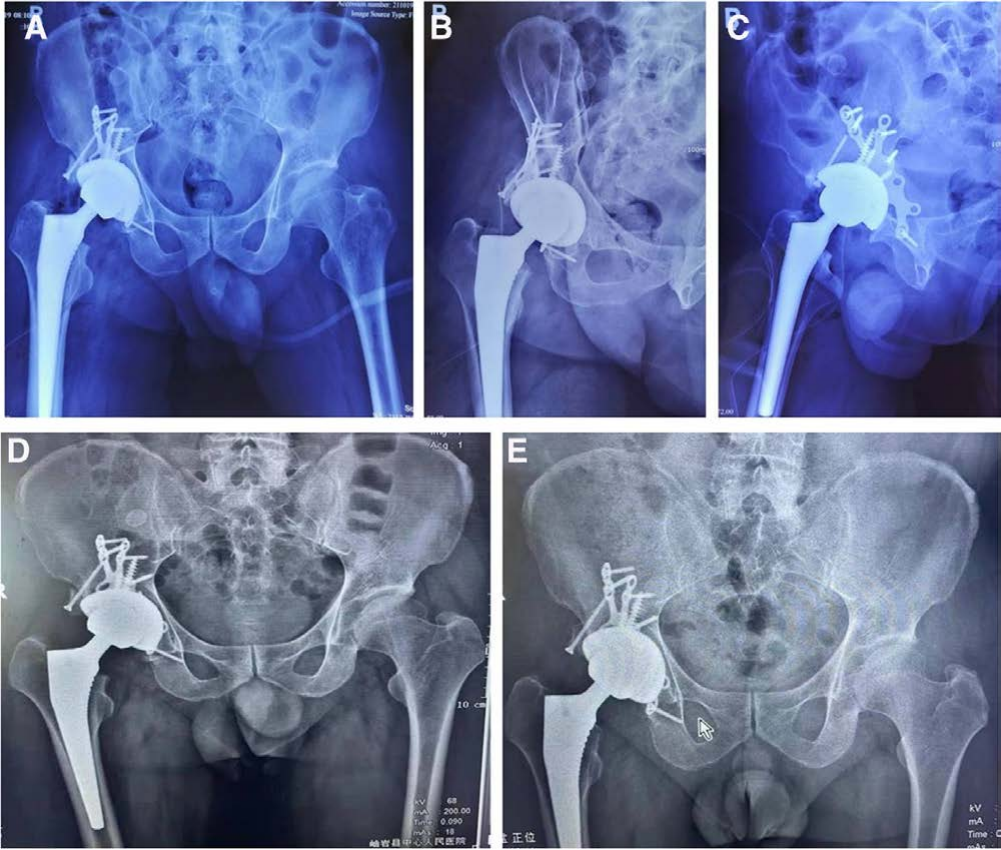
Postoperative X-ray images. (A–C) X-ray images of the patient right hip ORIF and THA 1 d postoperatively, including bilateral hip anteroposterior image, Obturator lateral image, and Iliac oblique image. (D) X-ray examination of the patient right ORIF and THA 1 mo postoperatively. (E) The X-ray shows that the posterior acetabular wall fracture has healed, and the prosthetic is in good position, 6 mo postoperatively. ORIF = open reduction and internal fixation, THA = total hip arthroplasty.
